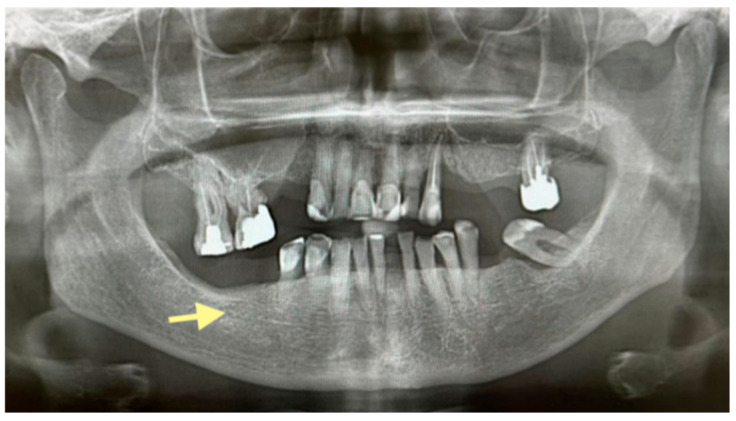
OPG (orthopantomogram) before surgery (12 January 2024). In the fourth quadrant, we do not see any changes, which are typical in the initial stages of osteonecrosis.
